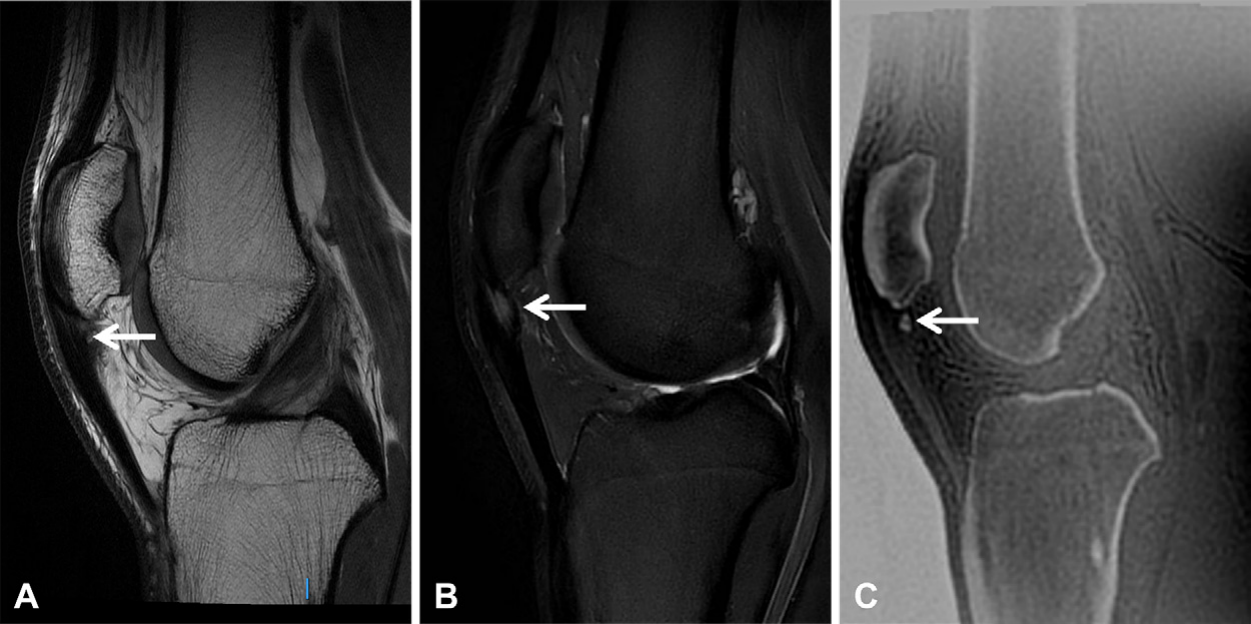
A 24-year-old high-level football player presenting with anterior knee pain, consistent with jumper’s knee. (A) Sagittal proton density-weighted and (B) T2 fat-suppressed images demonstrate focal thickening of the proximal patellar tendon insertion with increased intratendinous signal intensity (arrows). (C) The sagittal ZTE image depicts a small calcification (arrow) that is not appreciable on conventional MRI sequences.
